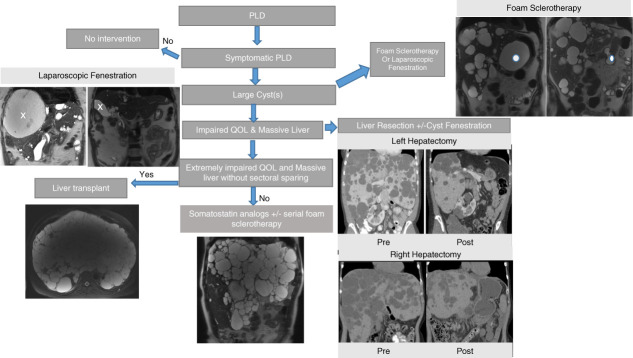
Algorithm for PLD management in ADPKD. Asymptomatic or mildly symptomatic patients may require no long-term intervention. Patients who develop impaired quality of life may benefit from foam sclerotherapy, laparoscopic fenestration, liver resection (partial hepatectomy), or liver transplant. Patients who are not candidates for surgical treatment may benefit from a trial of somatostatin analogs with or without serial foam sclerotherapy. Coronal T2-weighted MRI images are shown. The x and the dot show the changes in a cyst before and after therapy. ADPKD, autosomal dominant polycystic kidney disease.
